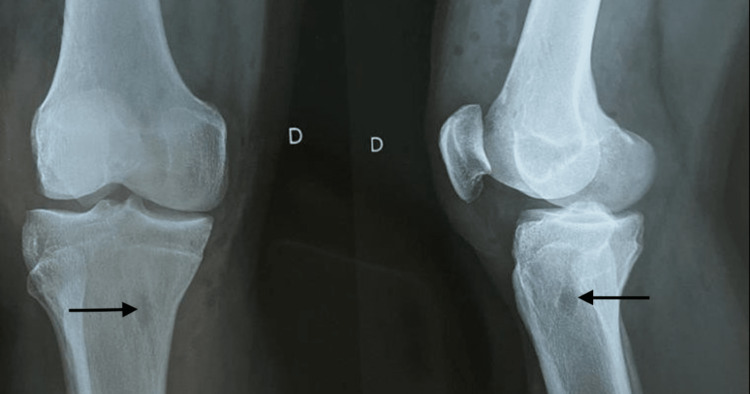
X-ray of the knee showing the tibial and femoral fixation using a screw
